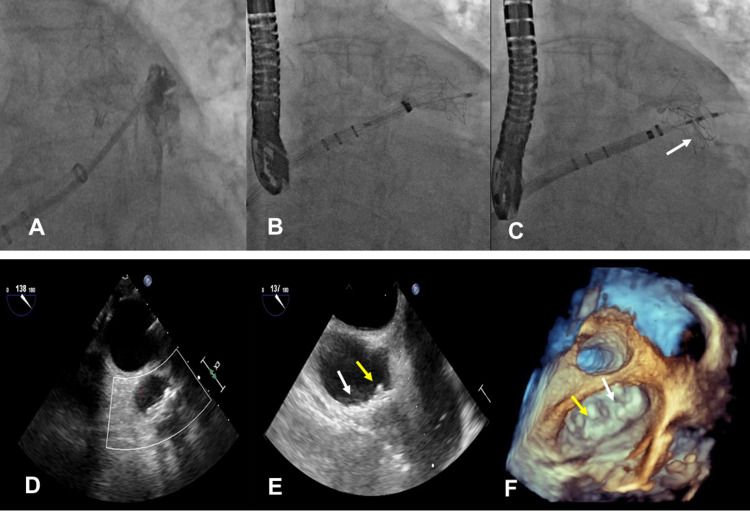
Percutaneous closure of moderate peri-device leak with Cardioform septal occluder (CSO) after initial Watchman implant Panel A: Angiographic examination of PDL in proximity to the Watchman occluder. Panels B-C: Fluoroscopic image showing delivery of the 25 mm Cardioform septal occluder within the PDL (arrow). Panels D-F: TEE showing closure of LAA and PDL with the Watchman occluder (yellow arrow) and CSO (white arrow), respectively. Panel F: 3D TEE en face showing both occluders. TEE: transesophageal echocardiography; LAA: left atrial appendage; PDL: peri-device leak
